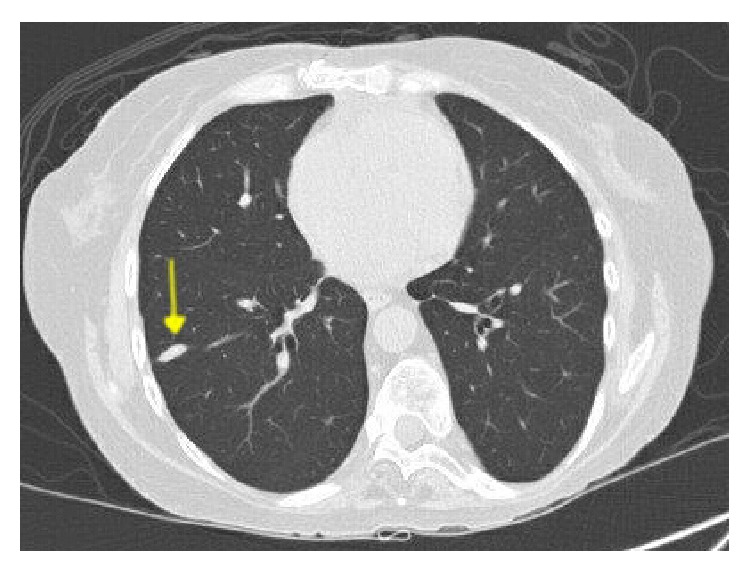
Computed tomography scan of the chest revealing a 19 × 8 mm nodule (arrow) involving the area of transbronchial biopsy on day 20 of the procedure.
